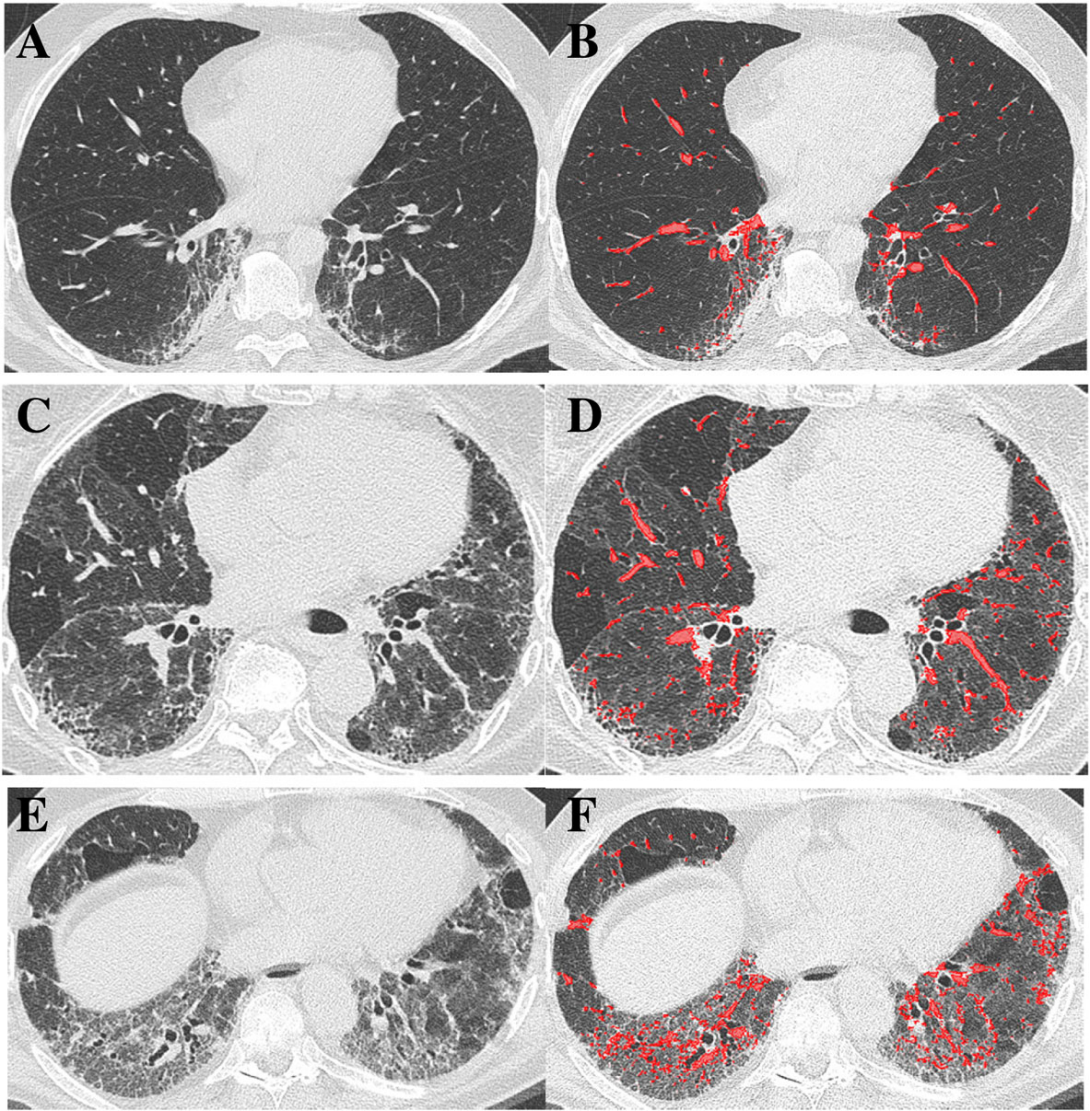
Corresponding axial CT images and colour overlay maps of CALIPER quantitation of pulmonary vessel volume (red), a volumetric measure of pulmonary arteries and veins within the lung, excluding vessels at the lung hilum in three chronic hypersensitivity pneumonitis patients with mild (a + b), moderate (c + d) and severe (e + f) extents of fibrosis. Note there is misclassification of small regions of the reticular pattern in the paravertebral regions (b) and right lower lobe (f) as vessels
